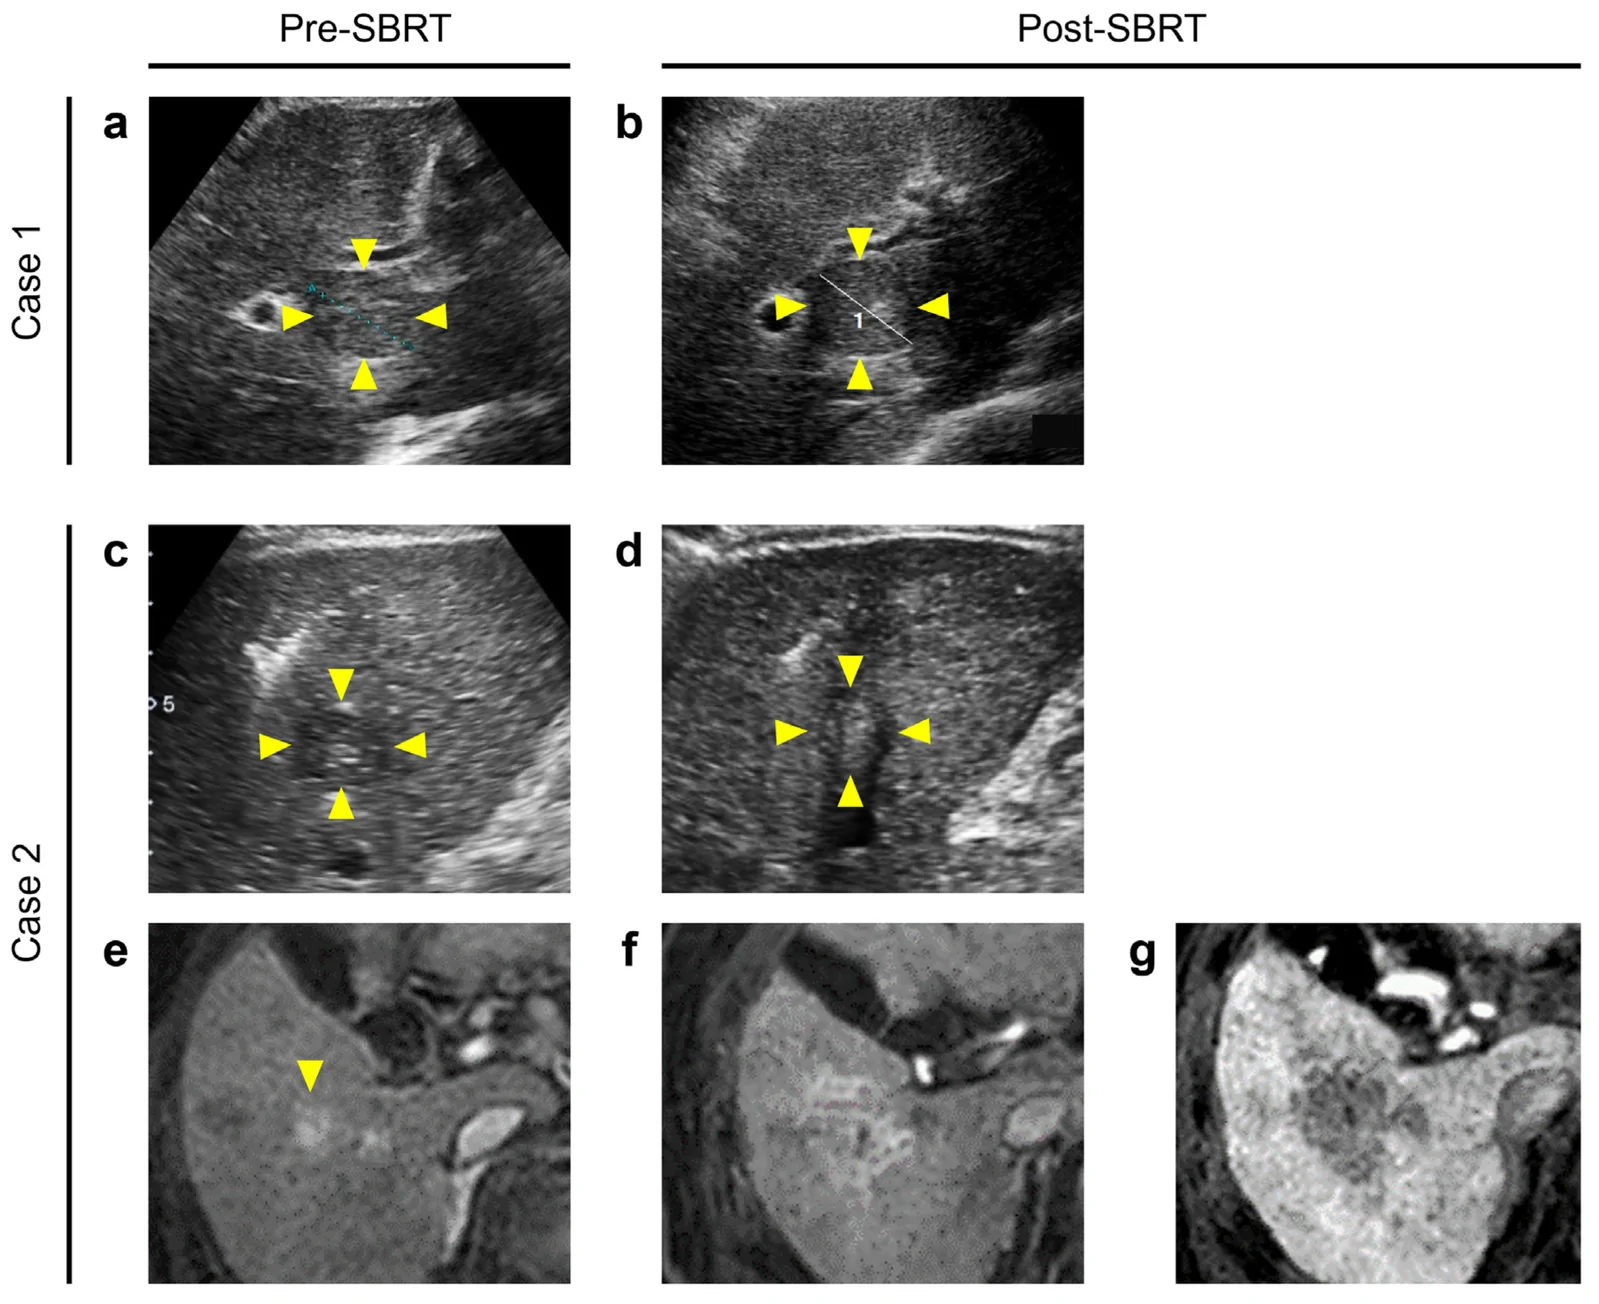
Representative cases of ultrasound (US) monitoring of tumor size after stereotactic body radiotherapy (SBRT). Images obtained before SBRT are shown in the left column (Pre-SBRT), and those obtained after SBRT are shown in the right column(s) (Post-SBRT). On the ultrasound images, the treated lesion is indicated by yellow arrowheads. Case 1 (a,b): An 87-year-old man with local tumor progression. On grayscale US, the maximum diameter of the treated nodule in segment 8 increased from 30 mm before SBRT (a) to 34 mm at 3.7 months after SBRT (b), preceding confirmation of progression on dynamic CT/MRI. Case 2 (c–g): A 60-year-old man with a treated nodule in segment 5. On grayscale US, the nodule remained stable in size after SBRT ((c) before SBRT; (d) after SBRT), whereas contrast-enhanced MRI showed persistent arterial-phase hyperenhancement (f) and hypointensity on the hepatobiliary phase (g) of the treated lesion after SBRT ((e) before SBRT, with the arrowhead indicating the tumor); these post-treatment findings did not indicate local recurrence but made assessment of tumor size on MRI difficult.
